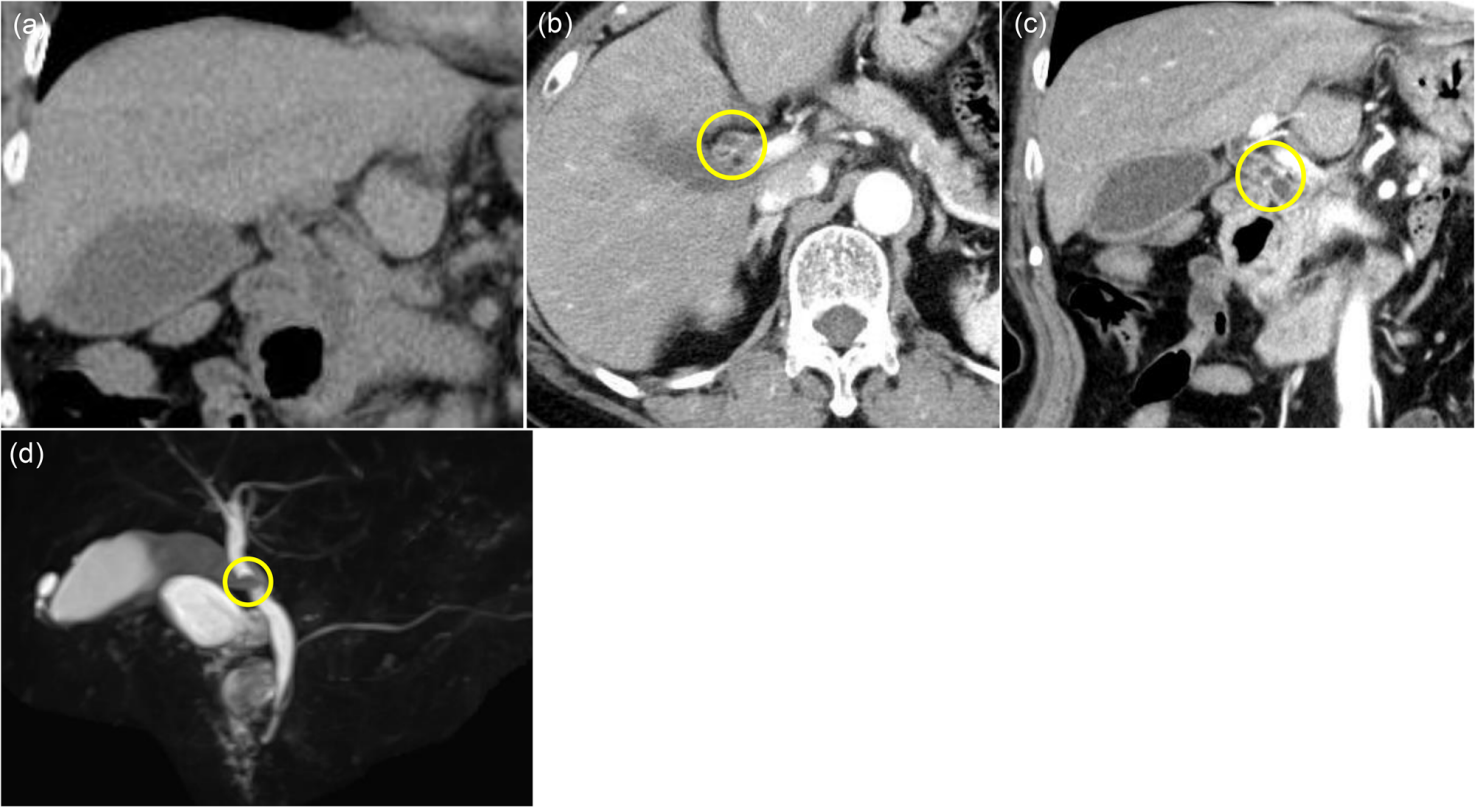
(a) Computed tomography. Common bile duct stones, gallbladder enlargements, and intrahepatic bile duct dilations were all visible and discovered on plain computed tomography. (b,c) Contrast‐enhanced computed tomography revealed thickening of the bile duct wall in the bile duct in the hilar region of the liver, and an enhancing effect is observed at the same location (yellow circle). (d) Magnetic resonance cholangiopancreatography revealed a lack of signal at the junction of the hilar region bile duct and the distal bile duct (yellow circle)
